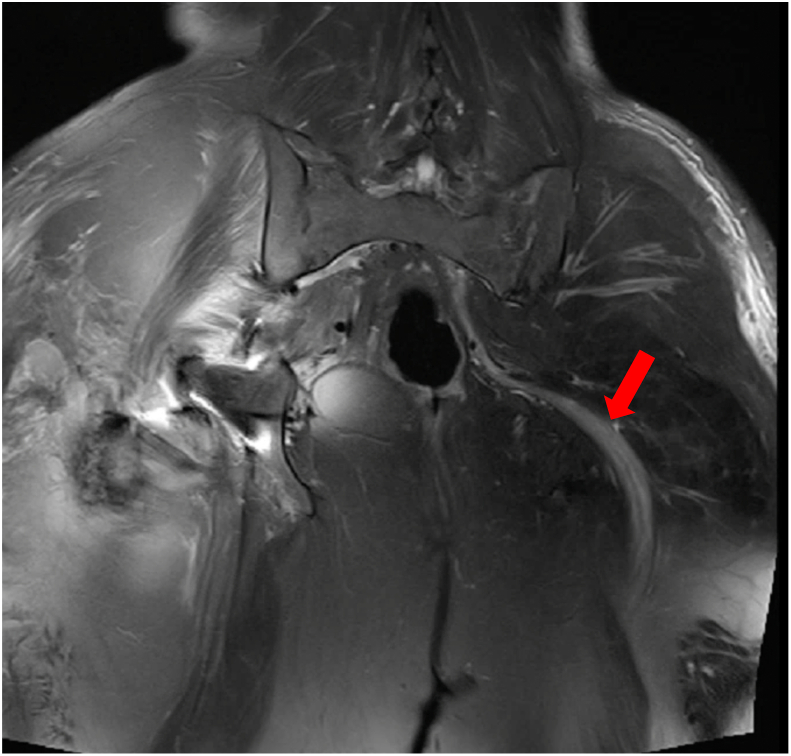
This MRI shows compression and inflammatory changes of the left sciatic nerve in the gluteal segment in T2WI.
